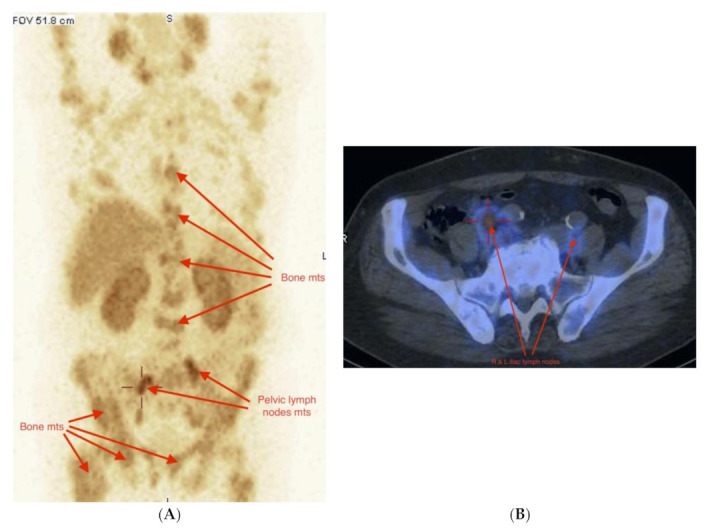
(A,B) Male 71 years, non-resectable PCa GlS = 9 (4 + 5), cTxN1M1, PSA = 7.2 ng/mL. During palliative hormonal and chemotherapy. Multiple bone mets and bilateral pelvic lymph nodes involvement in WB-SPECT/CT [^99m^Tc]Tc-PSMA-T4 evaluation of stage of disease. (A) WB-SPECT/CT, right and left enlarged pelvic lymph nodes and multiple bone mets in WB-SPECT (MIP) scan. (B) Osteosclerotic pelvic bone mets with pathological uptake of [^99m^Tc]Tc-PSMA-T4.
